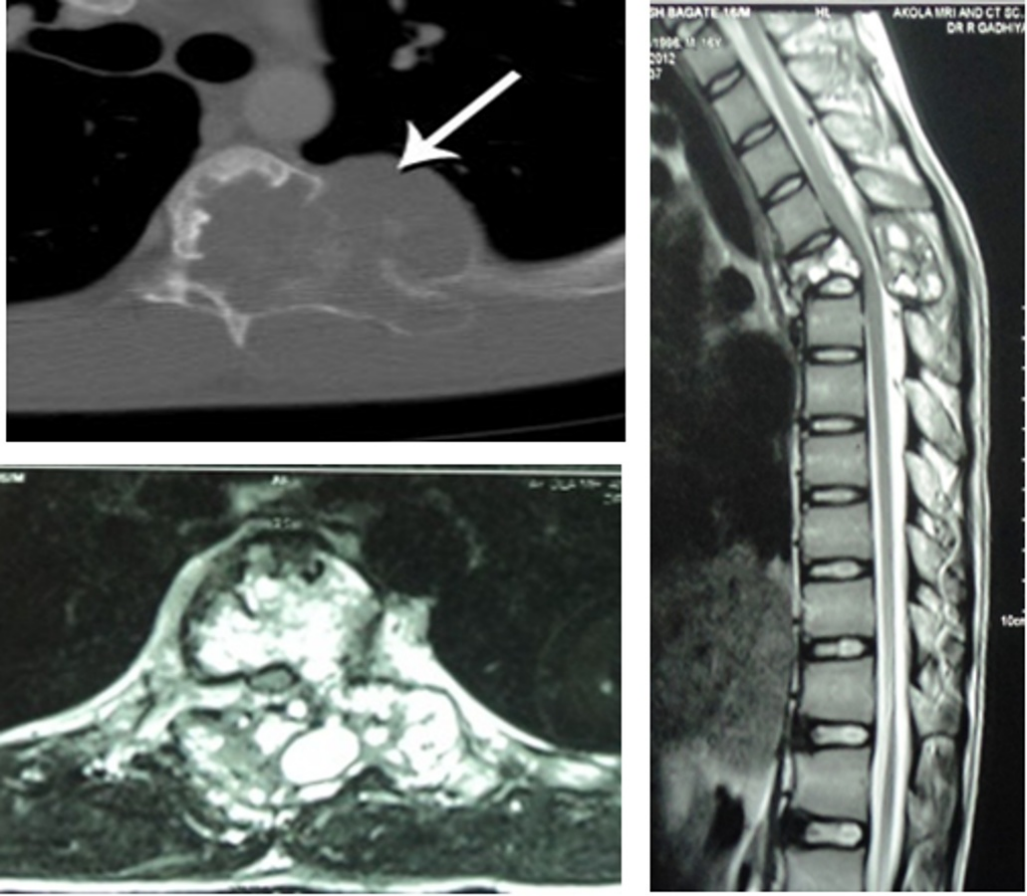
CT (top left) and MRI (bottom left and right) Computed tomography (CT), magnetic resonance imaging (MRI).
